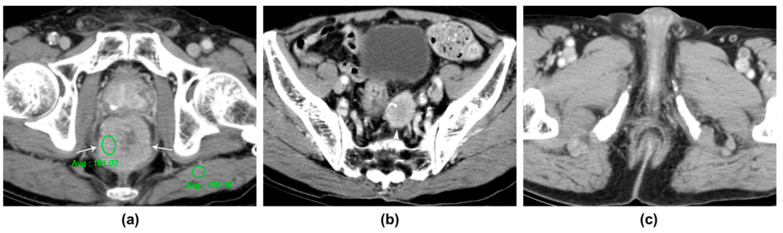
An 86-year-old man with squamous cell carcinoma of the rectum. (a) The contrast-enhanced portal venous phase CT image demonstrates homogeneously well-enhanced marked circumferential wall thickening (arrows) of the rectum. The ratio of tumor-to-back-muscle enhancement is 1.16 and is categorized as a moderate enhancement level (ratio between 1 and 1.5). (b) The second contrast-enhanced portal venous phase CT image depicts a large metastatic lymph node (arrowhead) with a spiculated margin and central necrosis (curved arrow) along the superior rectal vessel. (c) The third contrast-enhanced portal venous phase CT image reveals an intact anus without tumoral involvement.
